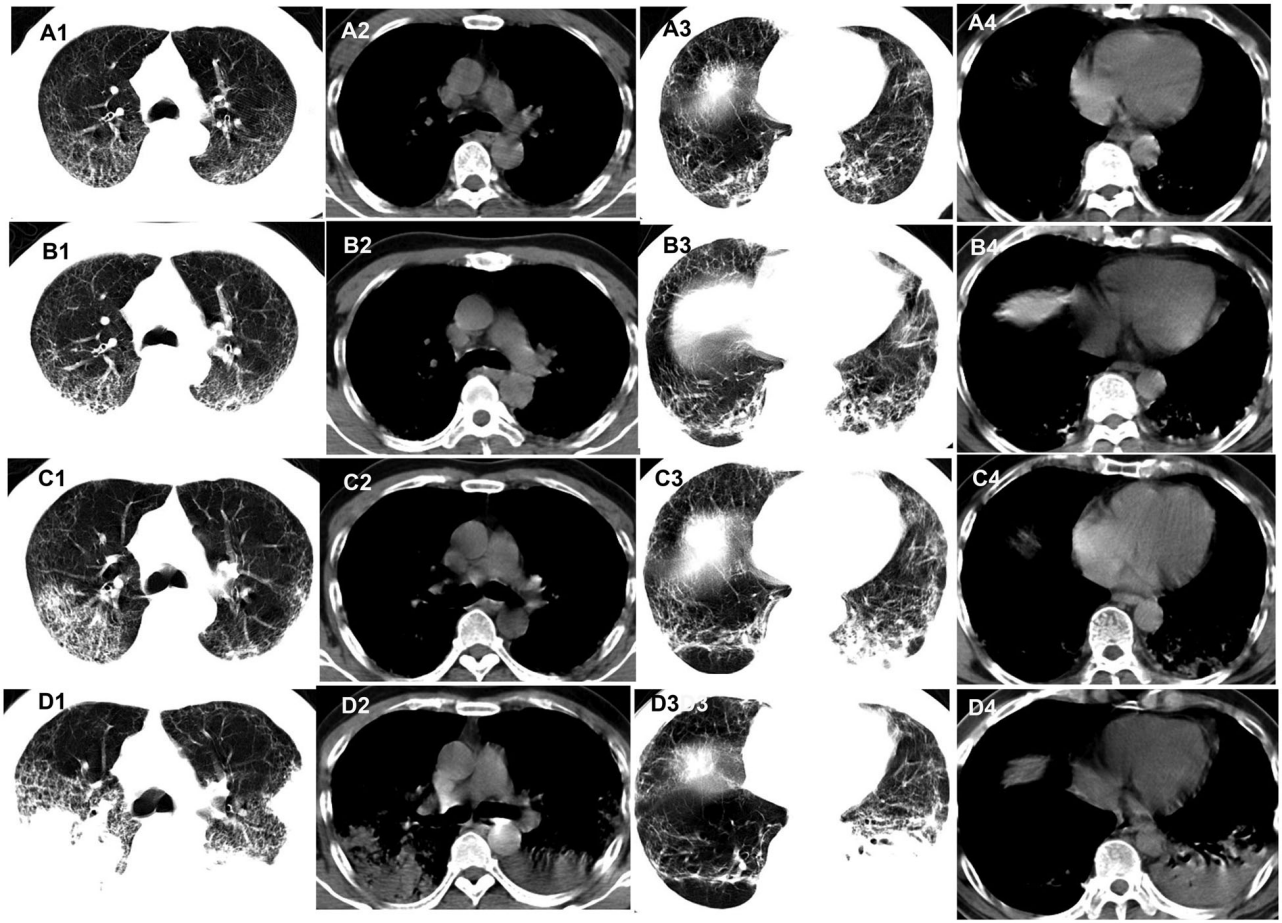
Evolution of the mixed pattern on chest CT of patients with COVID-19. A 64-year-old male Wuhan resident presented with cough, fever, and fatiguefor 3 days. (A1–A4) The first non-contrast-enhanced chest CT scan reveals multiple ground-glass opacities (GGO), fibrous stripes and visible interlobular septal thickening in the left and right upper and lower lobes (initial chest CT). (B1–B4) Follow-up chest CT 4 days after the first shows that both the scope and density of the lesions increase (stage I*). (C1–C4) Follow-up chest CT 8 days after the first shows that both the scope and density of the lesions increase further (stage II*). (D1–D4) Follow-up chest CT 12 days after the first shows that both the scope and density of the lesions increase even further (stage III*). *The stage does not represent the course of COVID-19 but the time interval between the two adjacent CT scans.
